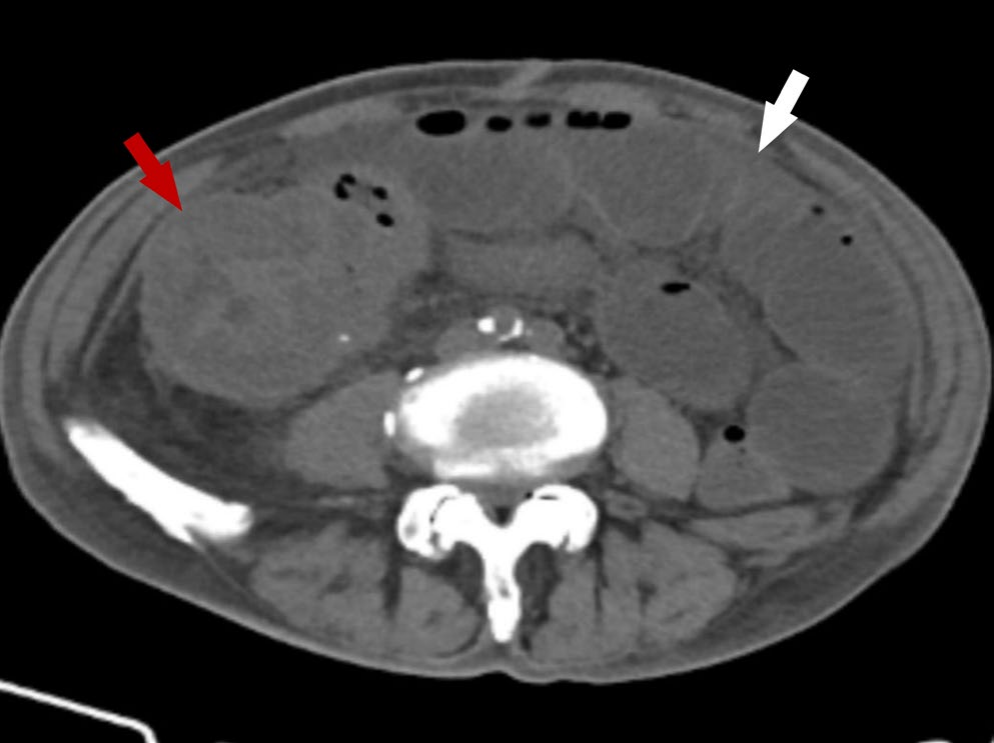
Preoperative computed tomography (CT) shows wall thickening in the ileocecal region and ascending colon (red arrowhead) with luminal narrowing, accompanied by proximal intestinal dilatation (white arrowhead).
